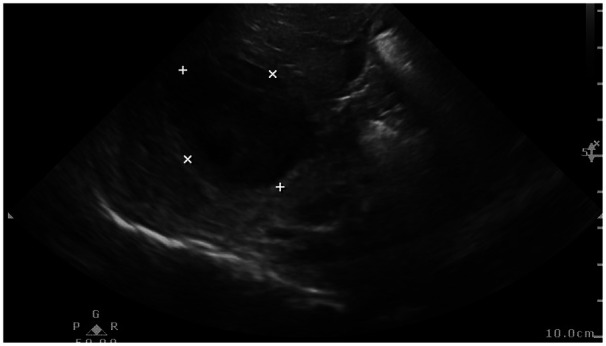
Abdominal ultrasound of patient 7 revealed a hypoechoic mass in liver segments S5 and S6, measuring approximately 42 × 33 × 54 mm. The mass exhibited well-defined borders, irregular internal walls, and areas of partial liquefaction appearing as dark regions.
